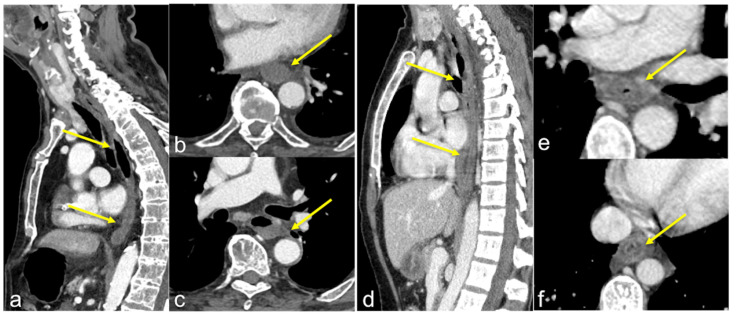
Two different patients with consequences of caustic ingestion on the oesophagus. Enhanced CTA scan in the portal venous phase in sagittal (a,d) and axial (b,c,e,f) views. Note, in the first case (a–c) the extensive oesophageal necrosis (a–c, arrows) with a thin and unenhanced wall, in the second case (d–f) the oesophageal thickening with a stratified wall (d–f, arrows).
